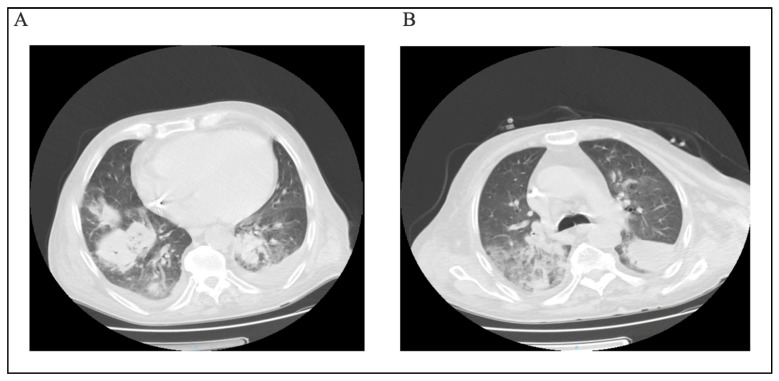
(A,B) CT scan section of patient with *Cryptococcus neoformans* infection.
